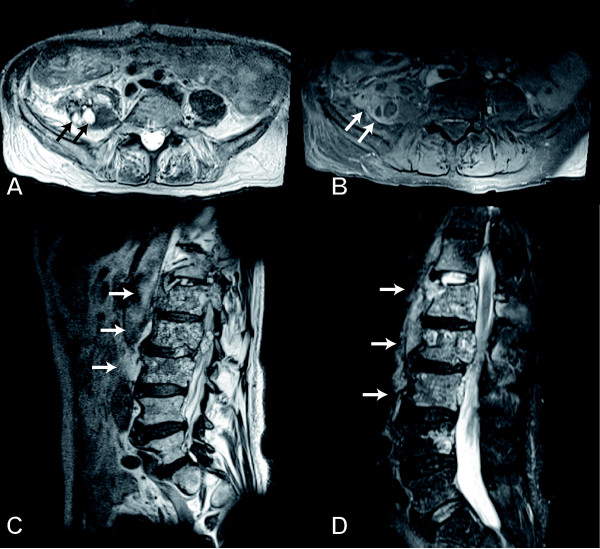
Magnetic Resonance Imaging of the lumbar spine. (A) The T2-weighted axial MR image shows multiple small hyperintense lesions in the right psoas muscle (arrows). The corresponding fat-suppressed T1-weighted image after gadolinium administration (B) confirmed the diagnosis of a multicystic mass and delineated the thickened, contrast-enhancing septations around the cystic components (arrow). T1-weighted image (C) and T2-weighted STIR image (D) of the lumbar spine in sagittal orientation. These images showed the bone marrow replacement within the first, second, and third lumbar vertebrae (arrows). The lesions comprise of cystic and solid components.
